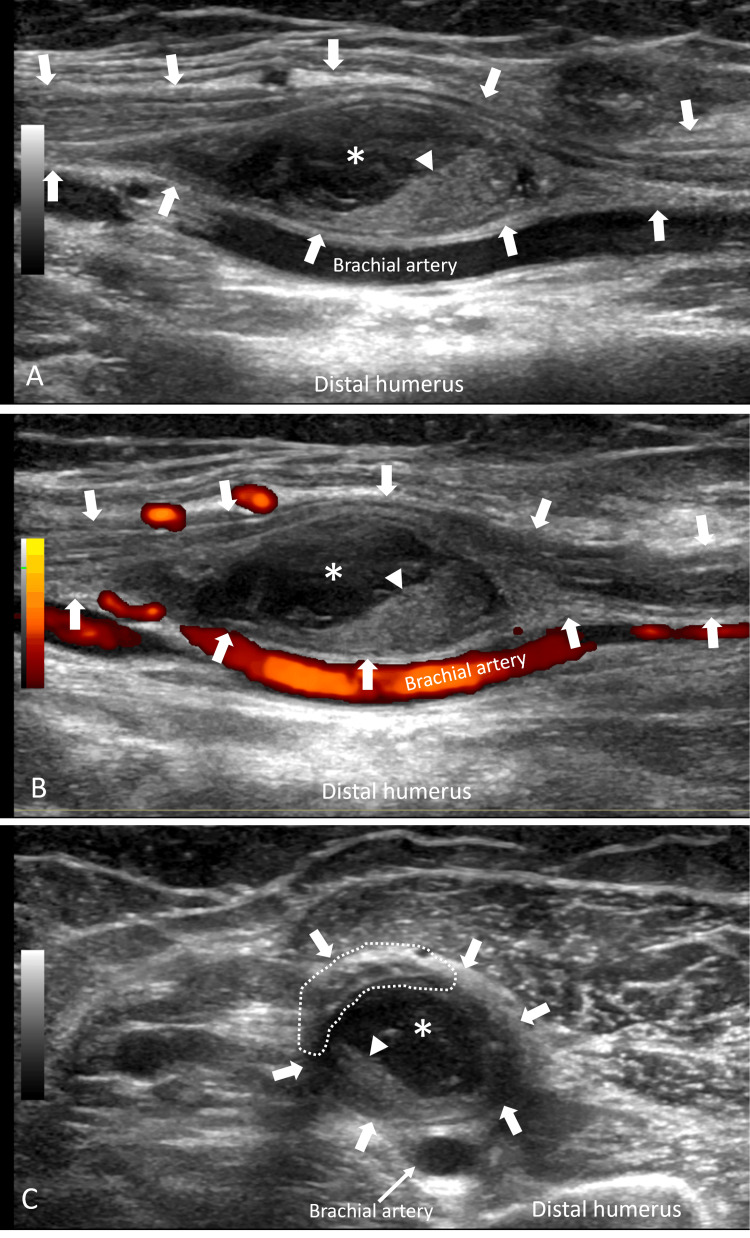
Median nerve ultrasound imaging in a 67-year-old woman with median neuropathy symptoms 2.5 months after traumatic PICC placement PICC: peripherally inserted central catheter Longitudinal grayscale (A) and power Doppler (B) US images and transverse grayscale (C) US image demonstrate a fusiform complex mass (*) encased within the median nerve epineurium (block arrows). The mass contains low-level echoes as well as echogenic eccentric tissue (arrowhead), features suggesting hematoma with internally retractile clot. The hematoma is encased in the epineurium but eccentrically displaces and compresses the nerve fascicles (dotted outline), consistent with subepineural position. Note the clear delineation of fascicular anatomy on longitudinal and transverse imaging
